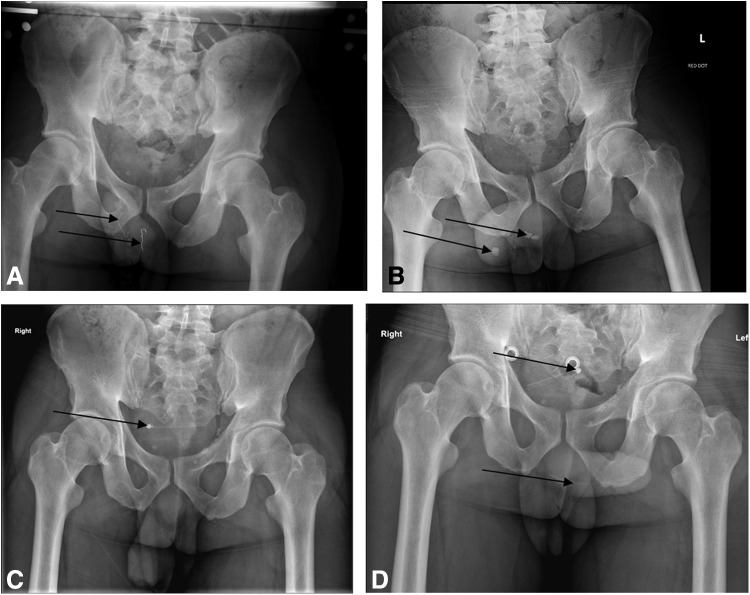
A selection of pelvic radiographs demonstrating FBs inserted into the patient's urethra, including parts of pens (B), paperclips (A), glass (B), and dismantled suprapubic catheter (C, D). FBs, foreign bodies.
